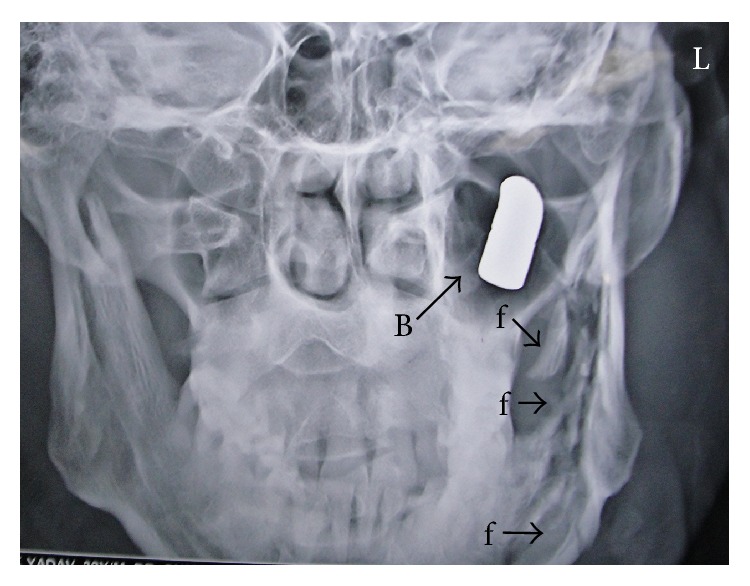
Preoperative X-ray of the skull, anteroposterior view, showing the bullet (B) lying in a sagittal plane passing through the middle of the left maxillary antrum. Note the multiple fracture fragments (f) of the mandible on the left side.
